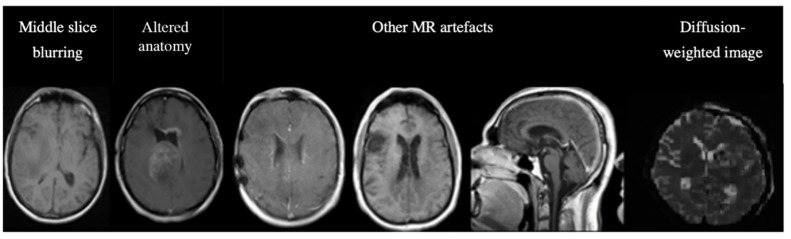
Examples of misclassified images. The first two images are examples of a misclassified MR, possibly due to blurry images (left) and alterations in expected anatomy (displaced ventricles, large tumor, right). The next three MR images show incorrect predictions due to different MR artifacts (shading, motion, aliasing). All of these images are falsely classified as “other”. The last image is a diffusion-weighted image (DWI), specifically a trace DWI, misclassified as T2w.
